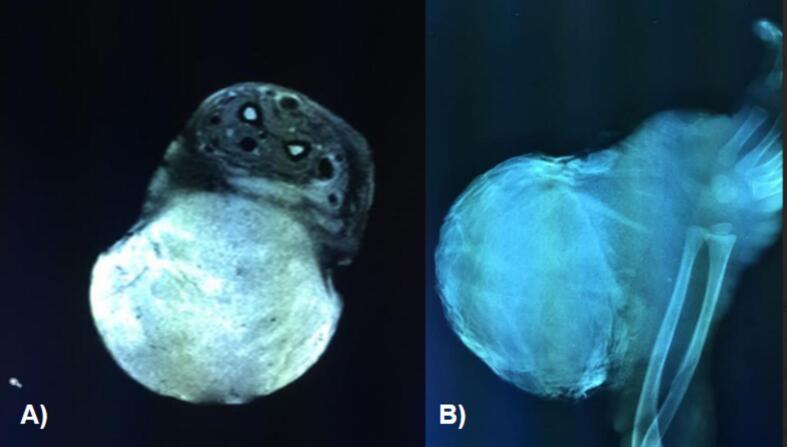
An axial section of a simple computed tomography of the massive heterogeneous tumor measuring 19 × 13 × 10 cm (A), no bone tissue involvement is observed, (B) plain radiography, lateral projection of the right upper extremity, tumor is observed in the right forearm, does not involve the humerus or radius, increased volume of soft tissues.
